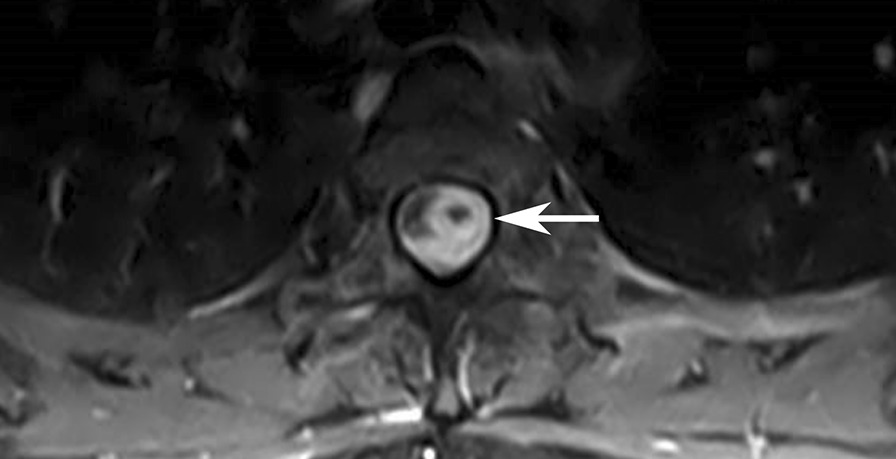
Magnetic resonance imaging showing a tuberculoma of the thoracic spine. Thoracic spine MRI showed an intradural extramedullary lesion at the level of T3/T4 invading the lateral aspect of the cord with severe mass effect and diffuse surrounding leptomeningeal enhancement throughout the thoracic spine. There was also high T2/STIR signal in the thoracic cord extending from T1-T7 levels concerning for cord edema
